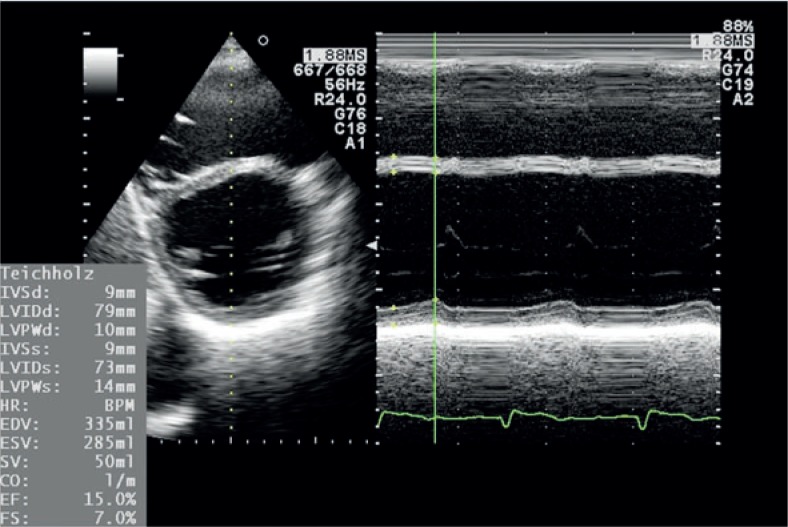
M mode measurement of left ventricular ejection fraction (LVEF) using the Teichholz method from the parasternal short axis view showing extremely enlarged left ventricle with severely reduced LVEF.
